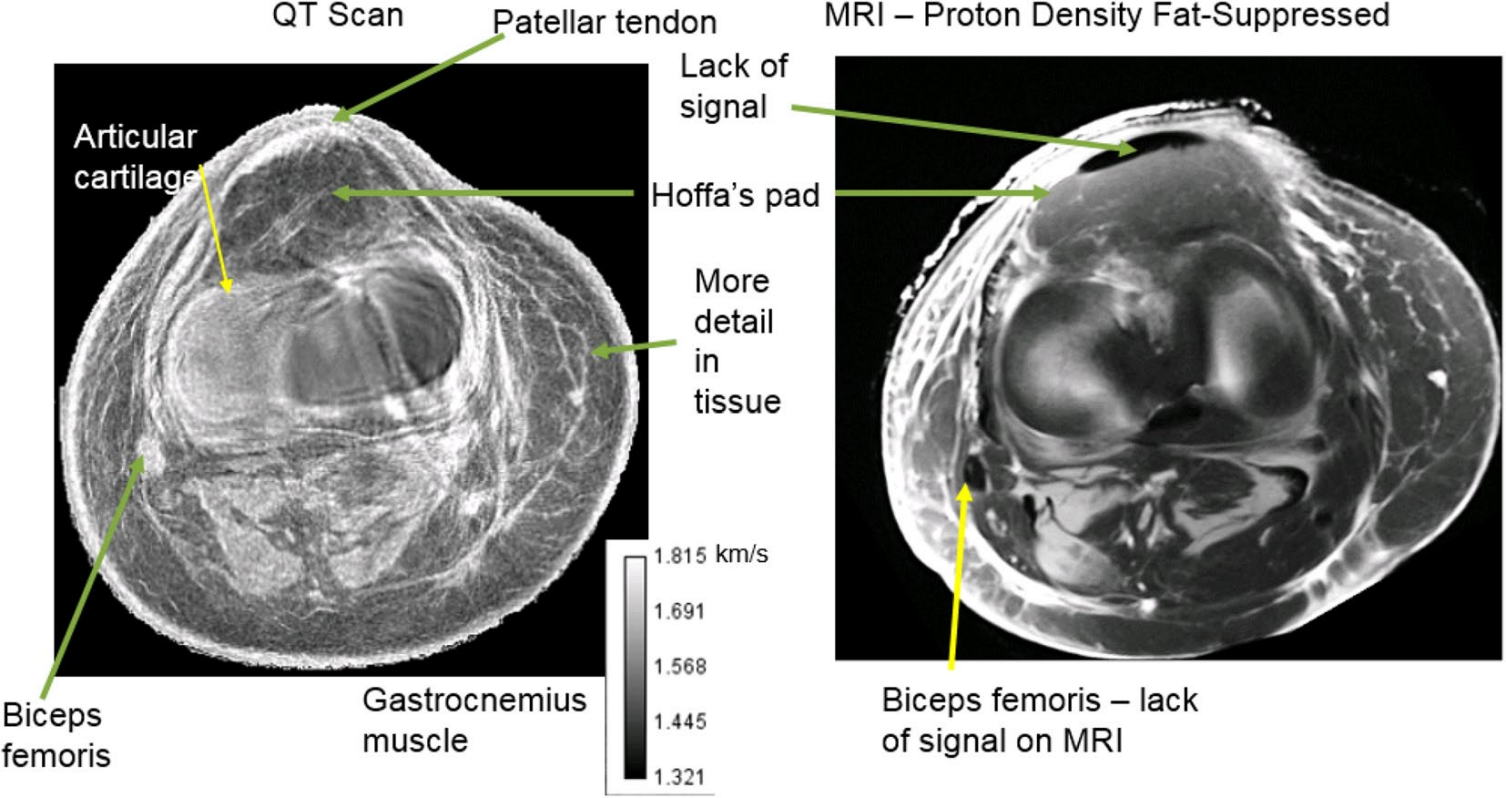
Comparison of the speed of sound image (left panel) with MR image of the same level. Note that the patellar tendon is invisible with this particular sequence for the MR image. The biceps femoris is also lacking signal from the MRI. Contrary to this, both the patellar tendon and biceps femoris have the correct quantitative speed of sound (high) for the SOS image. As noted in the text, there are structures in the QTUS image that are not visible in the MR image. We get quantitative values for the patellar tendon with QTUS, and no signal from the MR image, for example.
